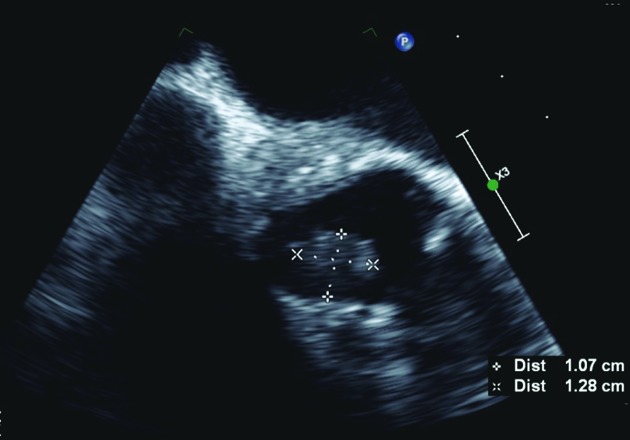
Transoesophageal echocardiograph of the bioprosthetic aortic valve.
